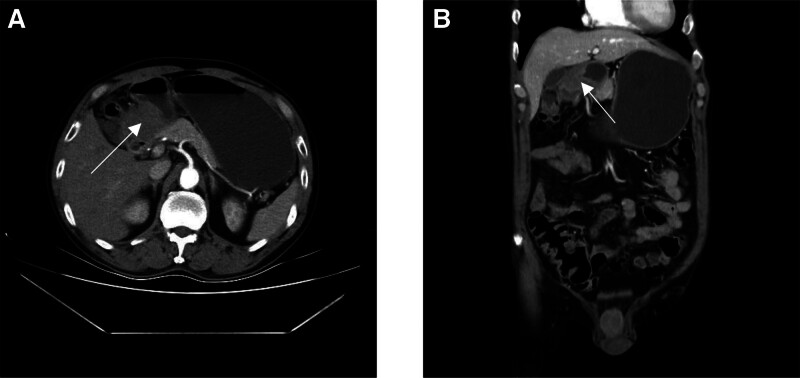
Contrast-enhanced CT scan after 3 cycles of neoadjuvant chemotherapy showed the diameter of the tumor reduced from 5.2 cm on admission to 4.2 cm (arrow). (A) Transverse section; (B) coronal position.
